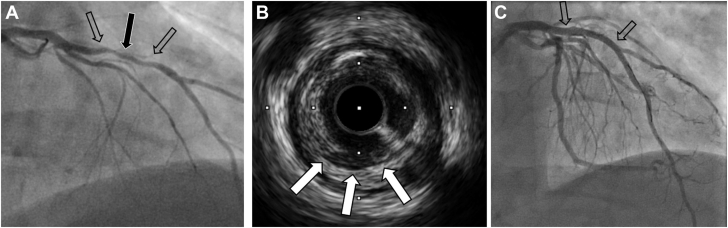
Coronary angiography—November 2024. (A) The obtuse marginal stent is patent; the LAD artery lesion progressed, both more stenotic (70%, open arrows) and with mid-lesion haziness (black arrow). (B) Intravascular ultrasound documenting mid-LAD plaque ulceration (white arrows). (C) Coronary angiography after successful stenting (open arrows). LAD, left anterior descending
